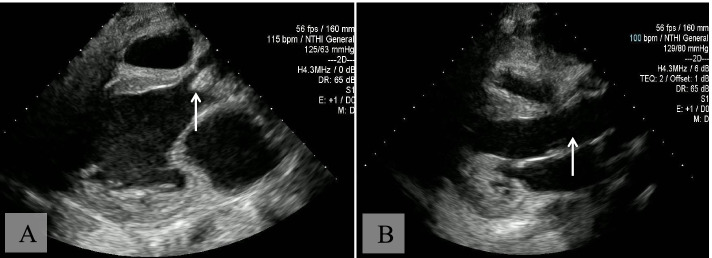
Echocardiogram. A Transthoracic echocardiogram, before surgery, two-dimensional, Doppler and color Doppler interrogation, parasternal long axis: Bicuspid and thickened aortic valve with vegetation (arrow), moderate to severe aortic valve regurgitation and mild to moderate aortic stenosis, mildly dilated left atrium and left ventricle with mild to moderate hypertrophy. B Transesophageal echocardiogram, after surgery, two-dimensional, Doppler and color Doppler interrogation: There is no left ventricular outflow tract obstruction. On-X mechanical valve shows no vegetation (arrow), with no significant stenosis or regurgitation. Left ventricle is mildly dilated with mild to moderately diminished systolic function
